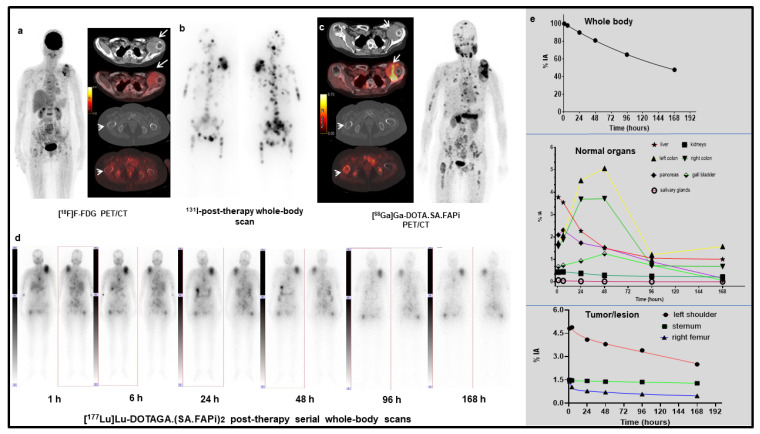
(a). [^18^F]F-Fluorodeoxyglucose (FDG) PET/CT images of a 50-year-old woman with follicular variant of papillary carcinoma, post radioiodine therapy (cumulative dose of 22.2 GBq) showing soft tissue density mass in left shoulder (arrows) and multiple skeletal lesions. (b). Whole body scintigraphy conducted after additional 7.4 GBq of radioiodine therapy, showing multiple foci of tracer accumulation suggestive of disease progression, who was started on Sorafenib (400 mg OD). (c). [^68^Ga]Ga- DOTA.SA.FAPi PET/CT images (performed after 6 months of Sorafenib therapy as part of ongoing clinical study when the patient had clinically progressive disease with thyroglobulin 300,000 ng/mL) show normal biodistribution in the oral mucosa, salivary glands, liver, pancreas, gall bladder, colon, and kidneys. Intense accumulation of radiotracer in the soft tissue mass (arrows) and multiple skeletal sites (right femur-arrow head). (d). Serial [^177^Lu]Lu-DOTAGA.(SA.FAPi)_2_ whole-body scintigraphy images for dosimetry, after intravenous injection of 1.48 GBq of radiotracer, showing radiotracer retention in the metastatic sites until 168 h delayed images. (e). Time–activity curves for whole body, organs that were easily discernible, and metastatic sites generated from region of interest placed on whole-body scintigraphy images. Accumulation in the normal organs peaked during 24–48 h and decreased significantly by 96 h post injection. Left shoulder, sternum, and right femur show persistent retention until 168 h delayed images. Patient received two cycles of [^177^Lu]Lu-DOTAGA.(SA.FAPi)_2_ therapy and showed significant clinical improvement with a decrease in thyroglobulin levels to 27,000 ng/mL. The patient also showed significant decrease in the VASmax score, from 10 to 5, in a follow-up at 4.5 months.
